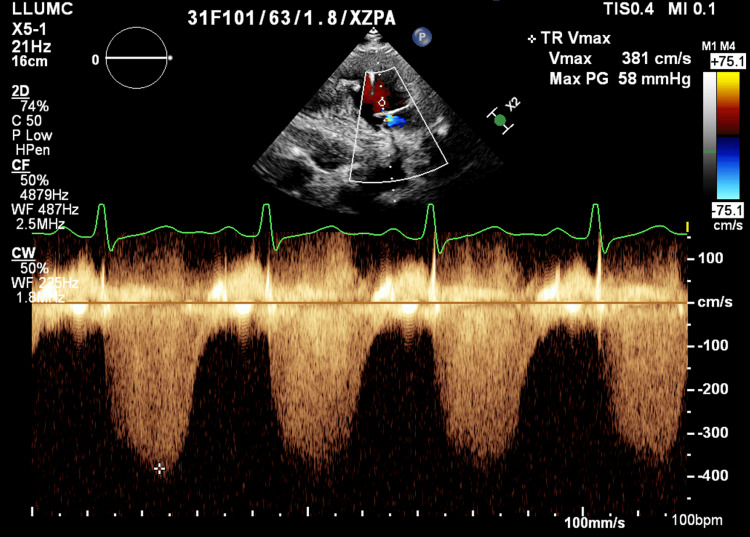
Transthoracic Echocardiography Estimating Pulmonary Artery Systolic Pressure TR Vmax: Tricuspid regurgitation max velocity; Max PG: pressure calculated by max velocity
